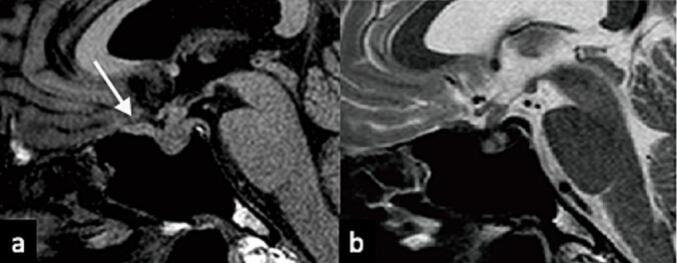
Initial image before treatment showing an intrasellar tumor with an extension to the dura mater (white arrow), cavernous sinus, and pituitary stalk. Sagittal T1-weighted image without contrast showing the isointense tumor without high signal in the posterior lobe (a). Sagittal T2-weighted image showing the isointense tumor (b).
